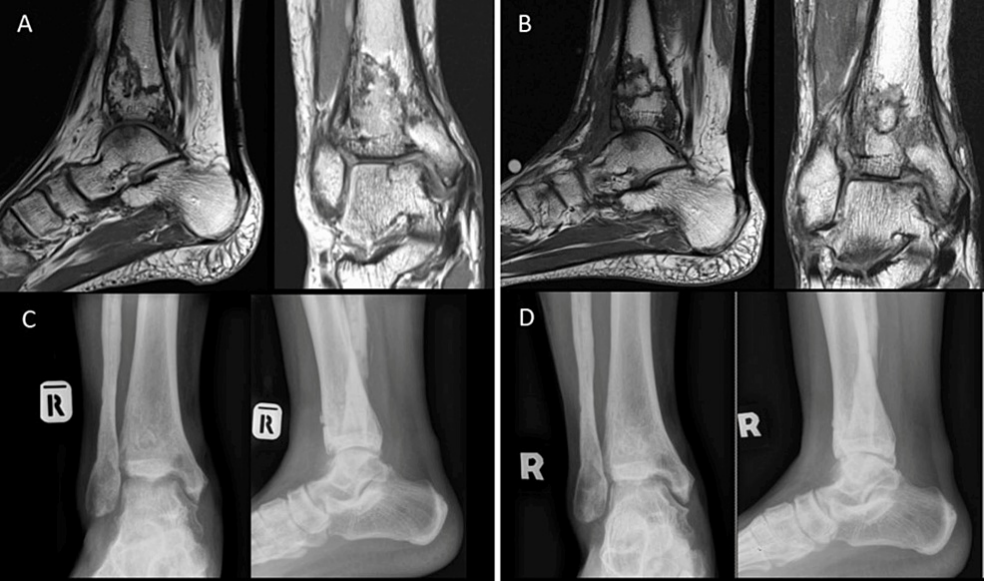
T1-weighted sagittal and coronal view (A). MRI image at one-year post-injury showed abnormal serpiginous signal intensity in the distal tibial metaphysis consistent with bony infarction. Repeated MRI study after six months post bone grafting revealed some area of bone healing (B). Radiograph of the ankle at six months post-surgery (D) showing healing of the bone window as compared to immediate postoperative radiograph (C).
